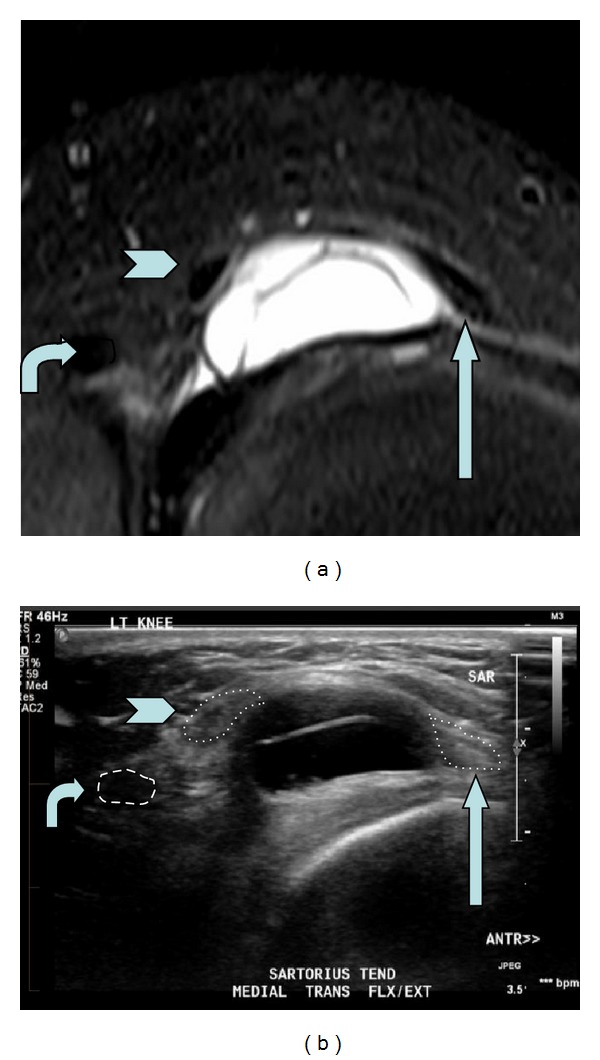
Comparison of (a) fat-saturated proton density MRI and (b) ultrasound left knee. Straight arrow: sartorius tendon; chevron: gracilis tendon; curved arrow: semitendinosus, with sartorius and gracilis “splayed apart” by parameniscal cyst.
